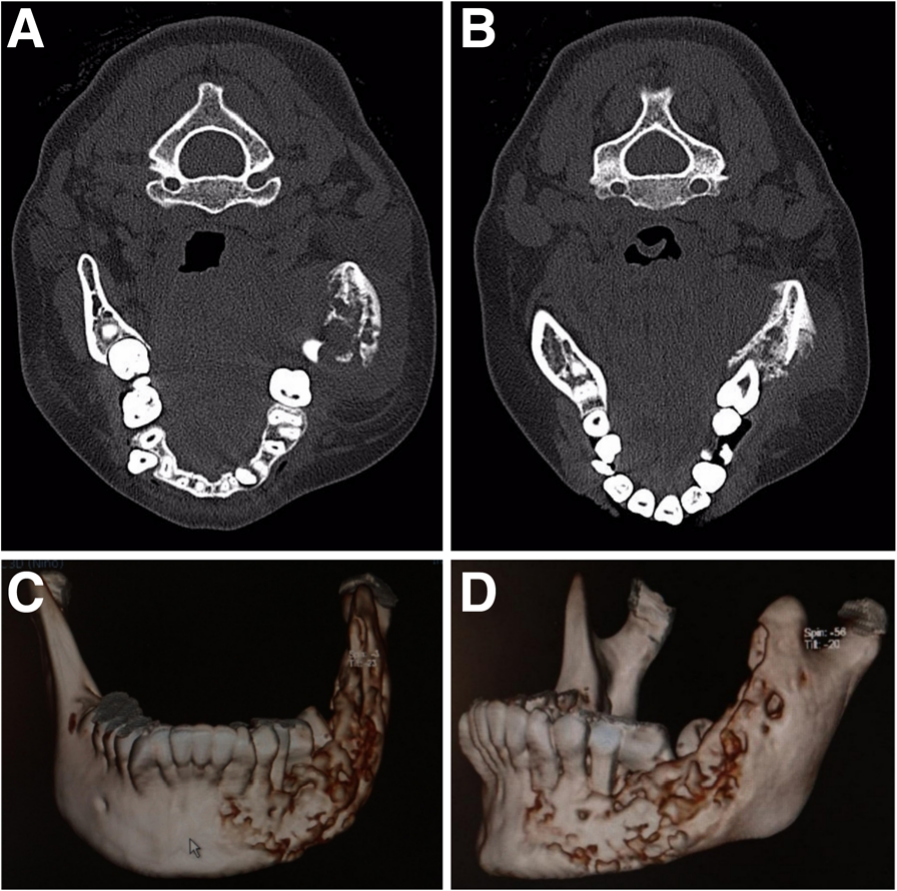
Clinical imaging of our patient with tuberculous osteomyelitis in the left mandible showing left-sided cheek swelling. (a) Frontal and (b) lateral views displaying mandible tuberculosis symptoms. (c) Frontal and (d) lateral views of three-dimensional reconstruction by computed tomography showing erosion and perforations of the cortical periosteal bone
